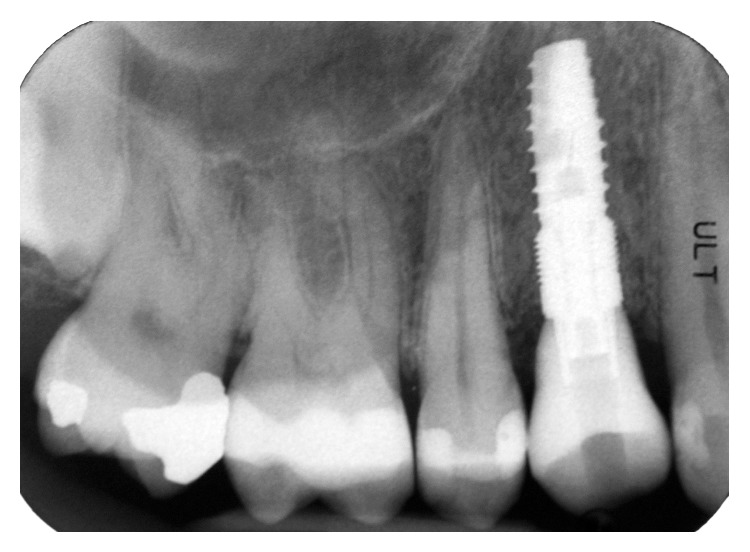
Radiographic view of the implant with the definitive crown screwed.
